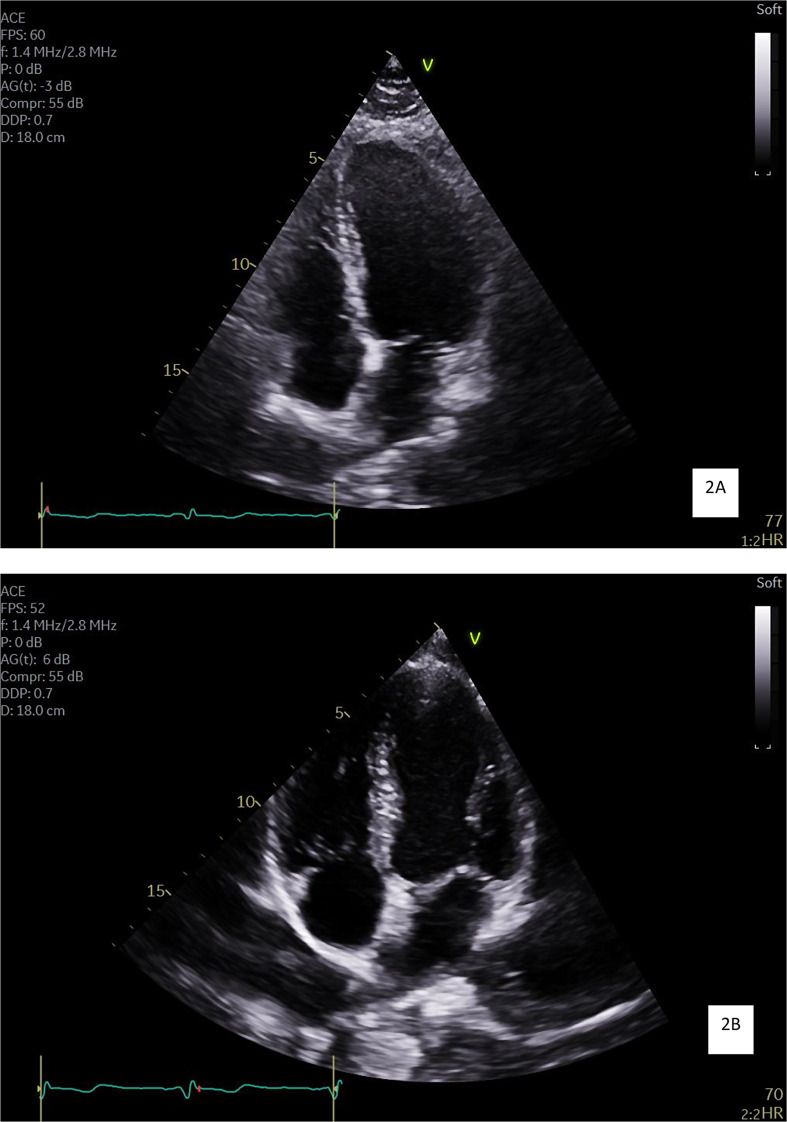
2A and 2B: Apical 4-chamber echocardiogram images demonstrating the chambers sizes in a general population participant (A) and jockey (B). Chronic high intensity physical activity results in dilation of cardiac chambers, particularly the left ventricle [22]
